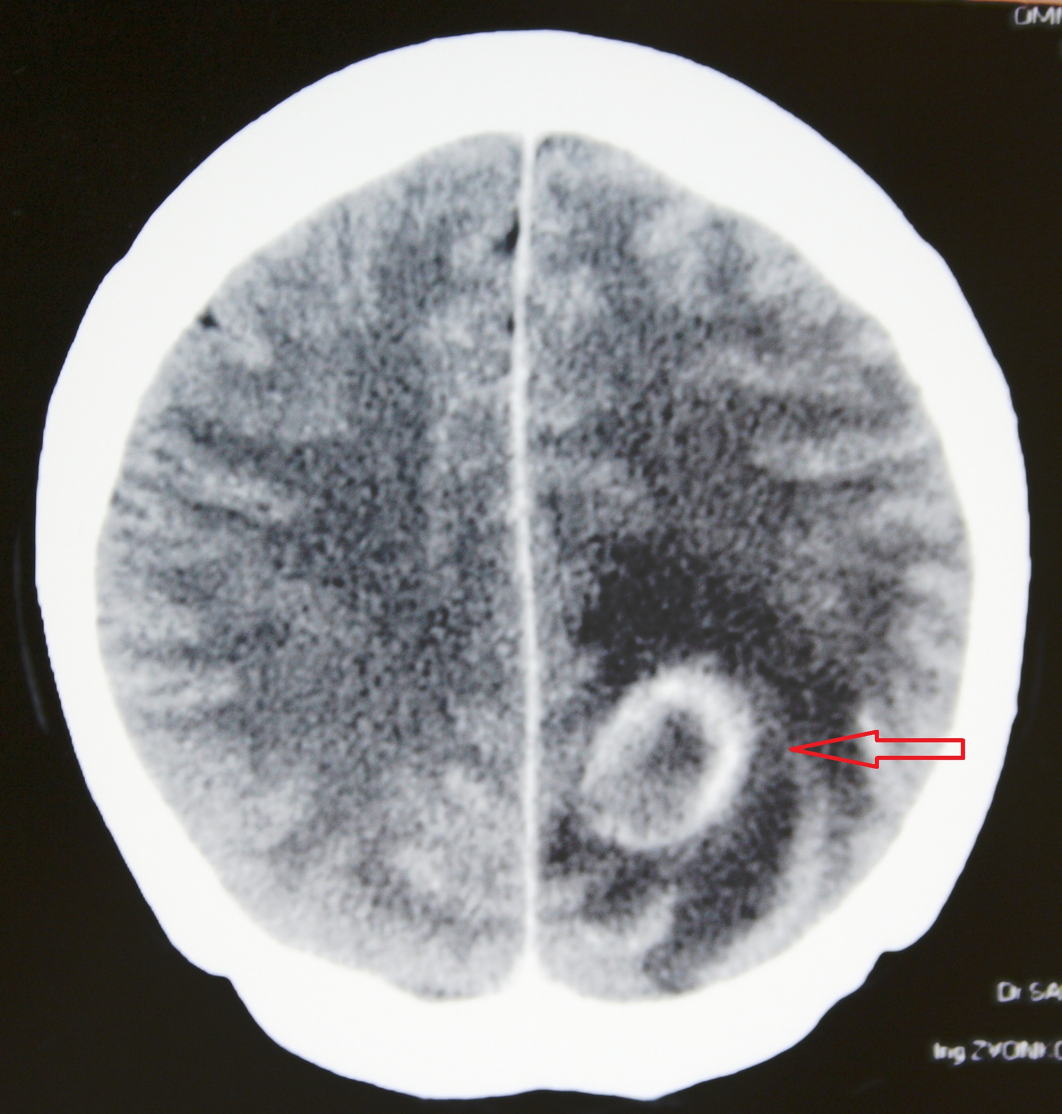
A CT scan of the brain with contrast medium obtained preoperatively revealed an expansive mass deeply in the left parieto-occipital region. A mass has the central zone of hypodensity surrounded by a ring of marked perifocal edema.
